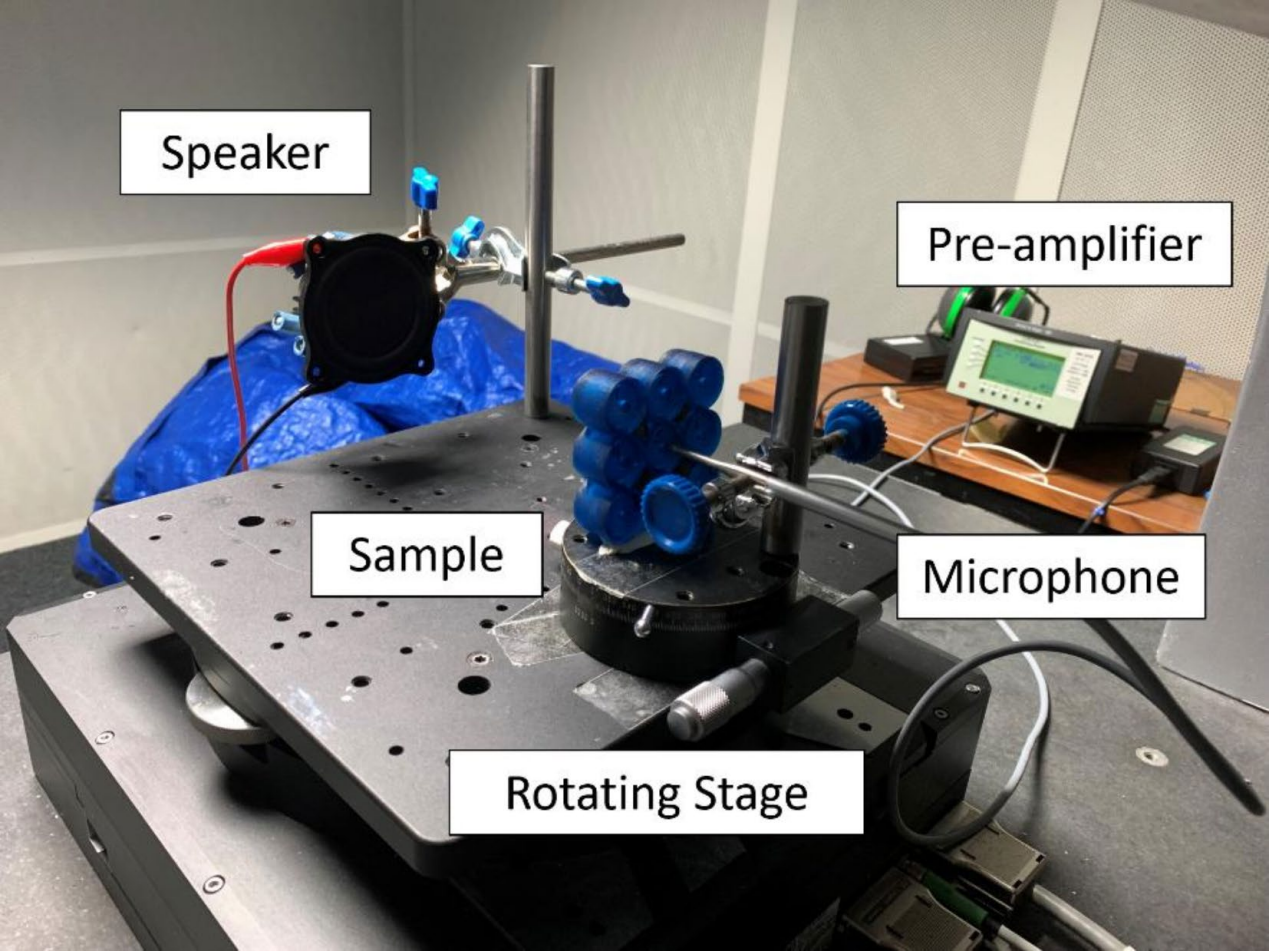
Experimental set up. The sample and the microphone are mounted on a rotating stage such that they both move relative to the speaker. The speaker and the sample-microphone system sit on an anti-vibration table inside an acoustic booth to minimise noise – particularly relevant at low frequencies. The speaker is connected to the laser Doppler vibrometer data acquisition system signal generator, and the microphone is connected to the pre-amplifier.
